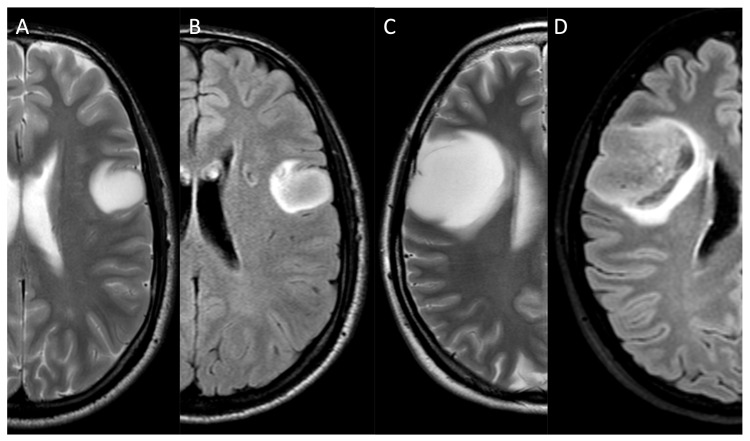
Shows the brain MRIs of two patients affected by LGGs. A 32-year-old man affected by an incidental grade 2, IDH-mutant diffuse astrocytoma involving the inferior and lateral (fronto-opercular) portion of the left frontal lobe. Axial T2WI (A) demonstrate a well-circumscribed hyperintense lesion, with the typical T2WI/FLAIR (A,B) mismatch. A 31-year-old woman affected by an incidental grade 2, IDH-mutant diffuse astrocytoma involving the fronto-opercular portion of the right frontal lobe (C,D). Axial T2WI (C) and FLAIR (D) sequences show a round lesion with the typical T2/FLAIR mismatch sign. *LGG: low-grade glioma; WI: weighted image; FLAIR: fluid-attenuated inversion recovery*.
